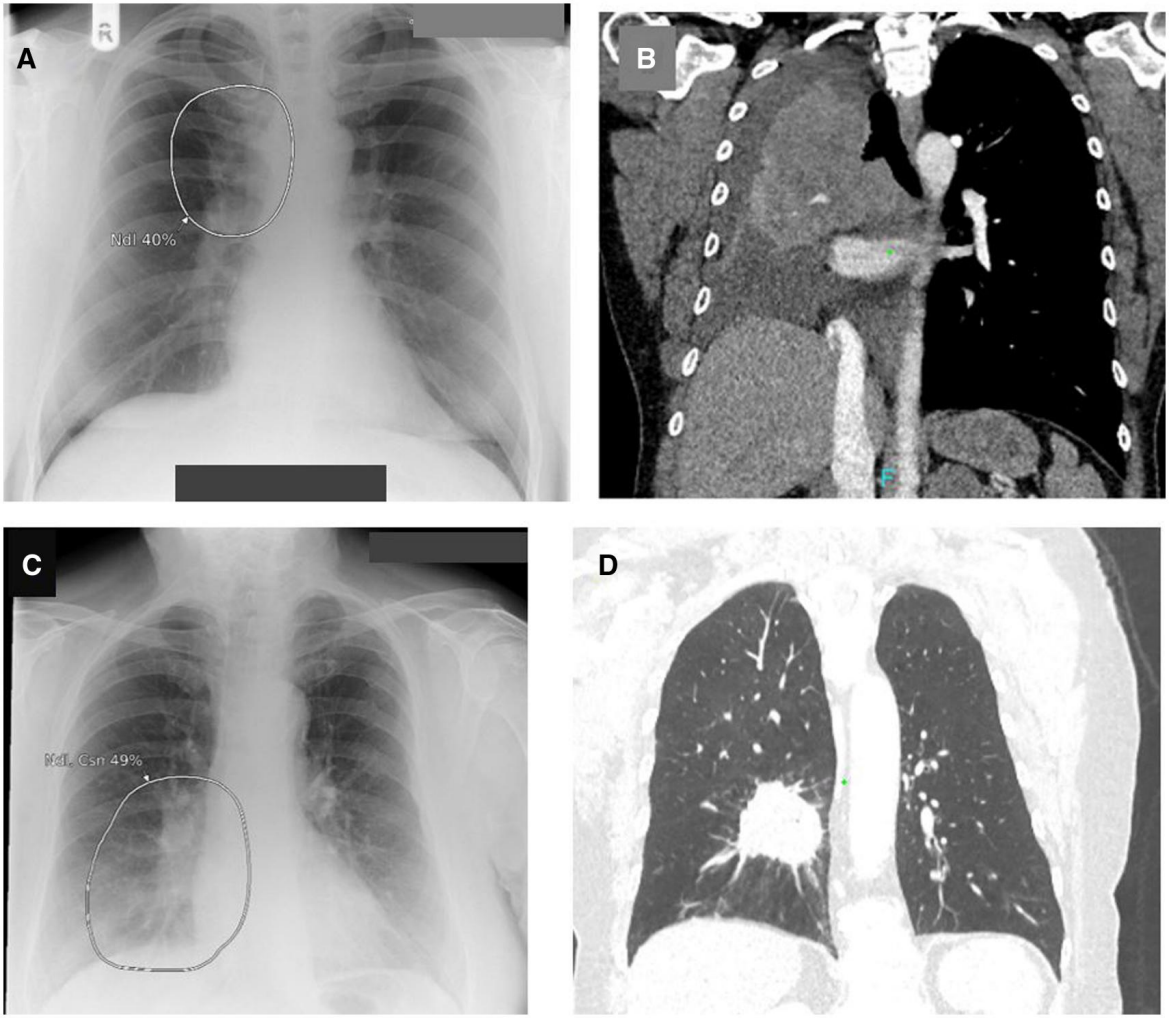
A 52-year-old male ex-smoker with a history of SOB and a cough underwent a CXR (A), reported as NAD. The AI software highlights suspicion of nodule (A, white circle) in the right perimediastinal region. One year and 1 month later, the patient attends for a CT scan (B) due to cough, SOB, and chest pain, which demonstrates a large mass confirmed as stage IV LC. An 87-year-old male smoker with history of COPD and a cough underwent a CXR (C) reported as NAD and *normal hilar shadows*. The AI software demonstrates suspicion of nodule (consolidation secondary) in the right hilar region. One year and 4 months later, the patient underwent a CT scan (D) due to an abnormal persistent cough, wheezing and phlegm that was not responding to antibiotics; this demonstrates a right hilar mass confirmed as stage IV LC. AI = artificial intelligence; CXR = chest radiograph; LC = lung cancer; NAD = no abnormility detected; SOB = shortness of breath.
